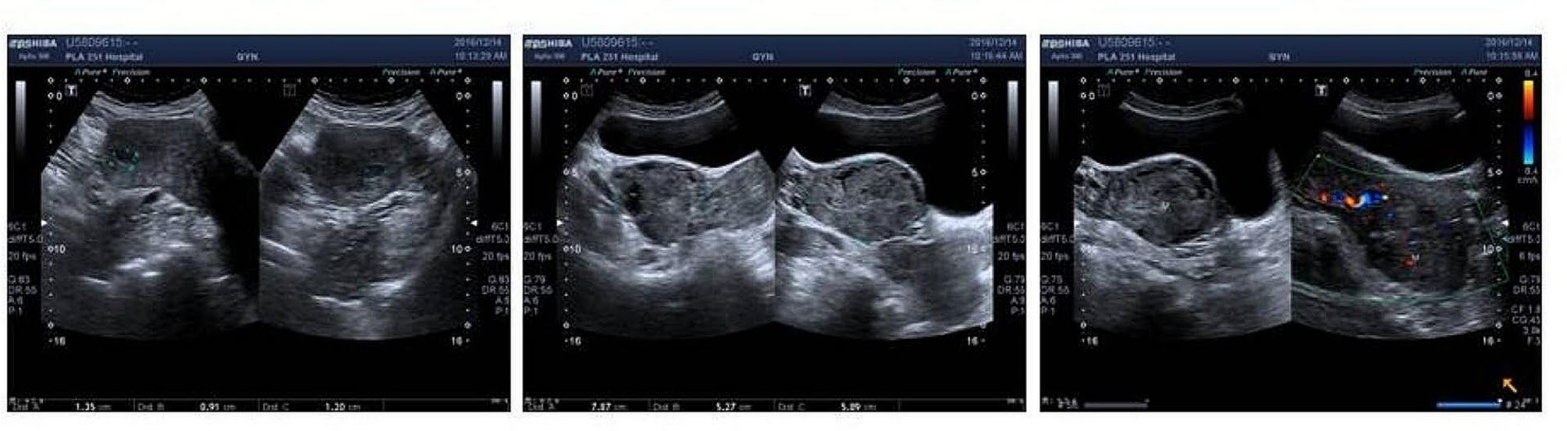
Pelvic ultrasonography identified obviously enlarged uterine mass, with a diameter of 13 cm
